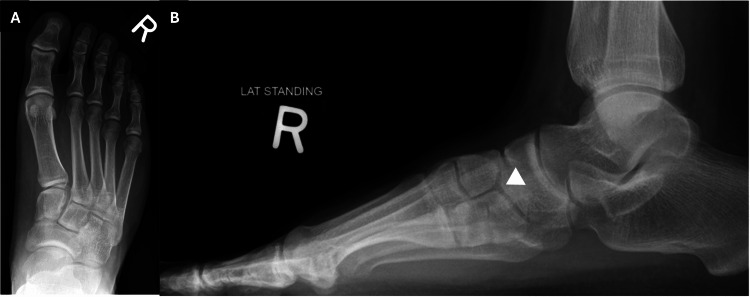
Weight-bearing radiographs of the right foot in anteroposterior view (A) and lateral view (B) showing bipartite medial cuneiform (white arrowhead).
